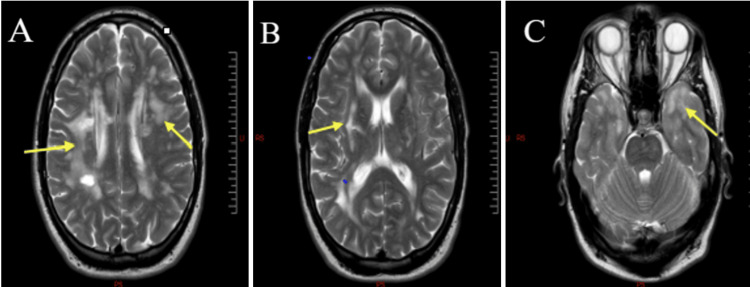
Axial T2-weighted MRI image of the index patient Presence of focal and confluent hyperintense lesions located bilaterally in the periventricular region (yellow arrows) (A), characteristic high-signal intensity of the external capsule (yellow arrow) (B), and anterior temporal pole (yellow arrow) (C)
